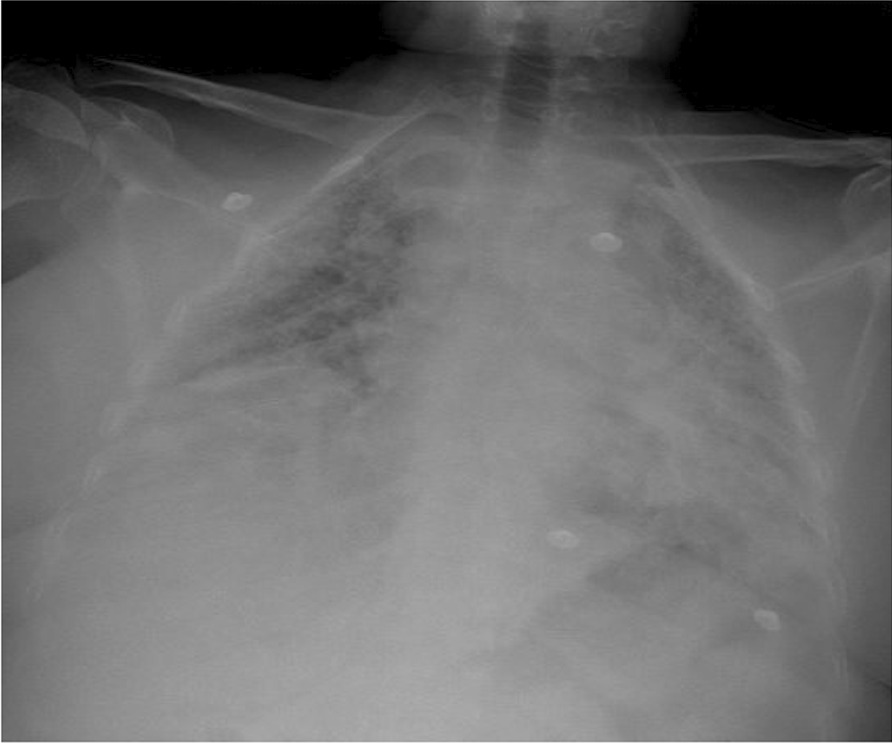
Repeated chest X-ray at day 21, showing worsening infiltrates
